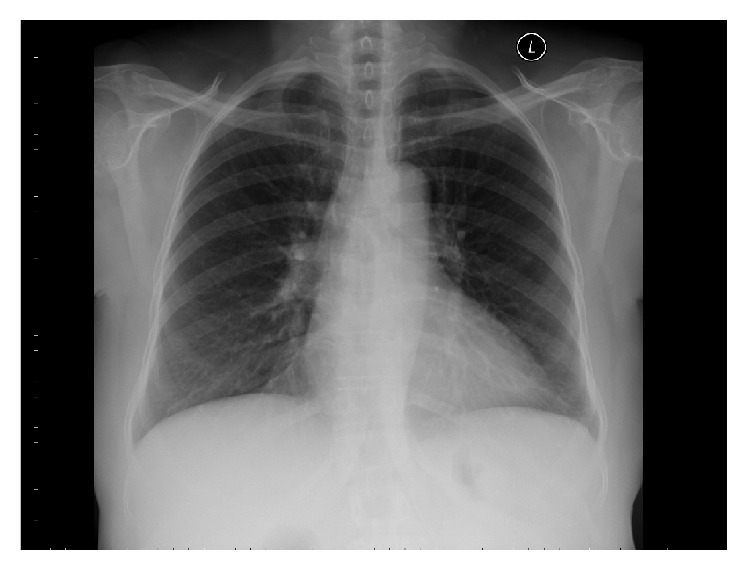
Chest X-ray at 12-month followup with complete resolution of miliary pulmonary nodules.
